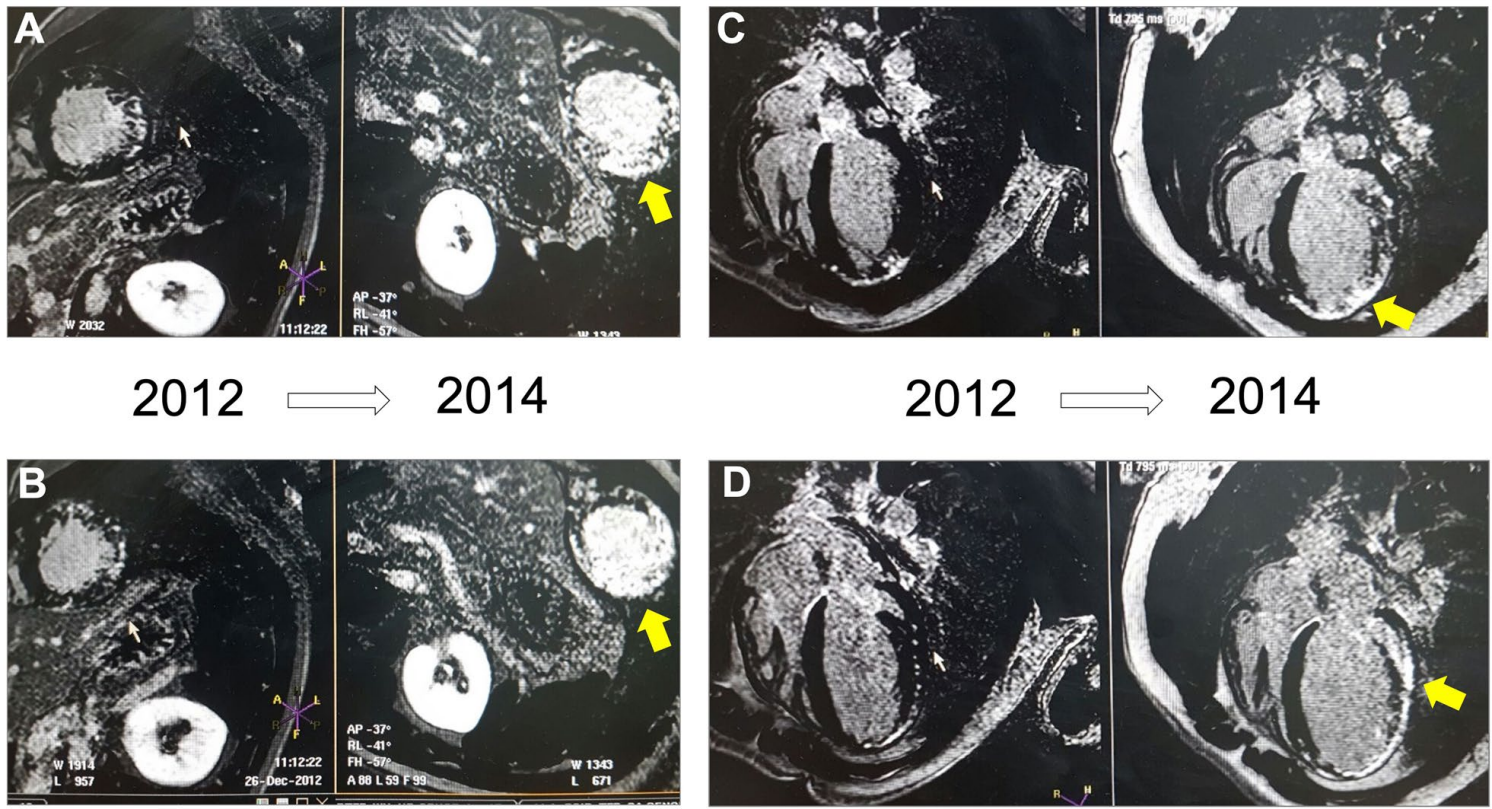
Massive myocardial loss in an adolescent patient with mutated PLKHM2 and familial cardiomyopathy. Sequential cardiac MRI studies; in all pictures left side shows an image from a 2012 exam and right side shows similar images from a 2014 exam. Late gadolinium enhancement imaging using phase-sensitive inversion recovery protocol (PSIR) is demonstrated. A, B short axis views, C, D four chamber views. Note focal patches of late gadolinium enhancement with prominent worsening in the second exams (yellow arrows). The patient was implanted with an ICD at the age 17.5 years, but had worsening heart failure and eventually died in hospital during preparations for cardiac transplantation. A skin biopsy obtained during ICD implantation was used to generate iPSCs that are examined in the present study (DCM-iPSCs, see text for details)
